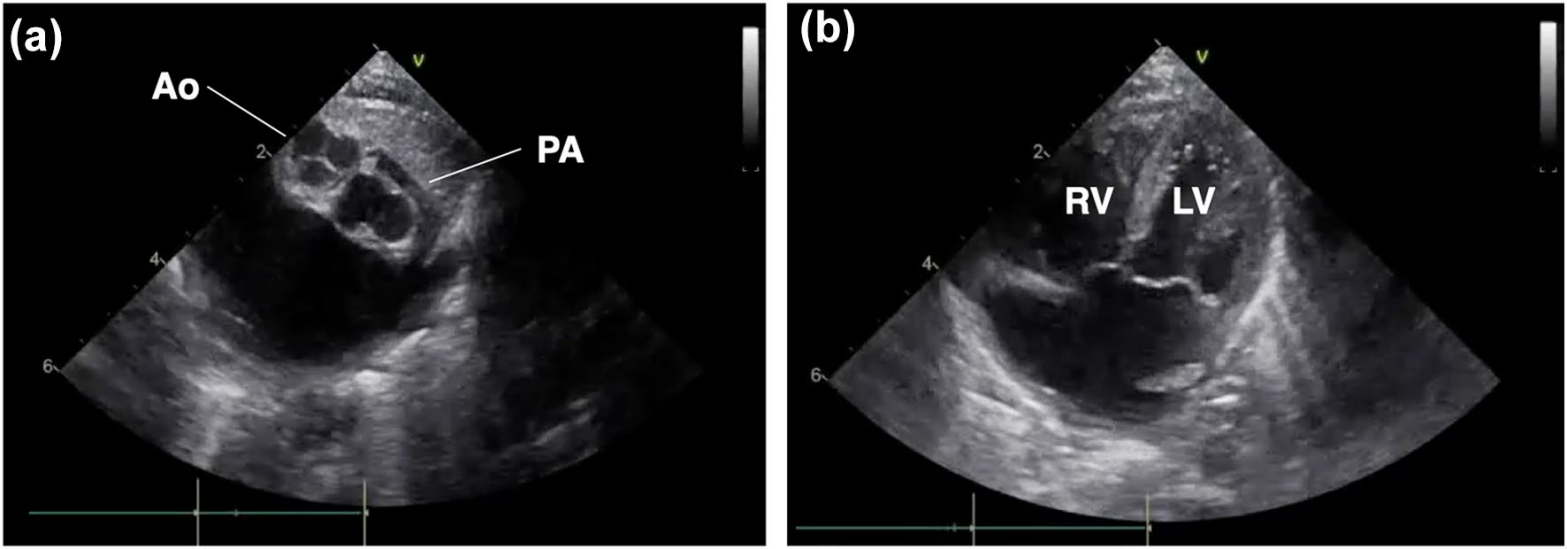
Preoperative echocardiography imaging. a The aorta was positioned right anterior, and the pulmonary artery was positioned left posterior; *Ao* aorta, *PA* pulmonary artery. b Four-chamber view showing balanced ventricles; *LV* left ventricle, *RV* right ventricle
